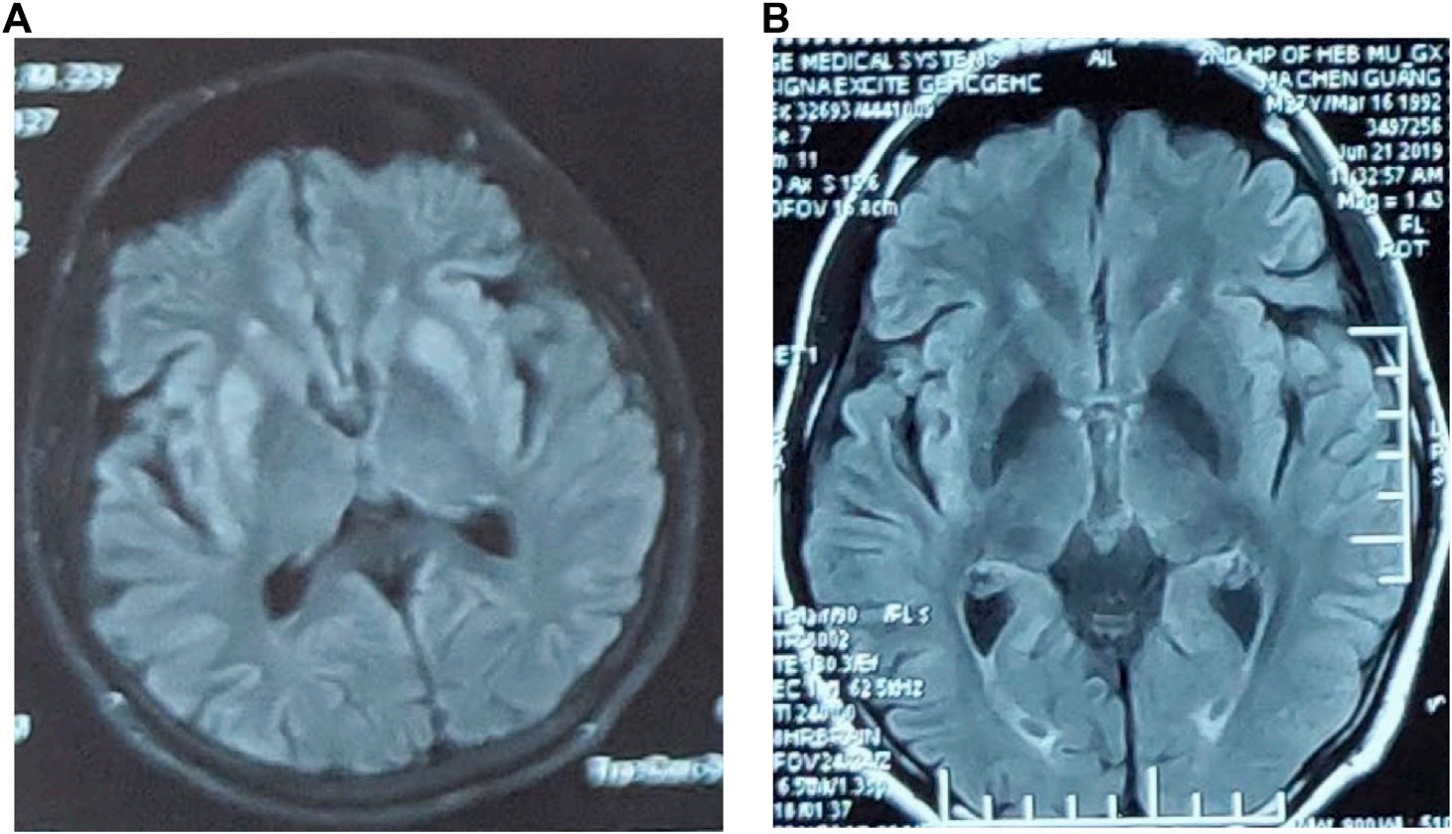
(A) Brain MRI showed symmetrical abnormal signals in bilateral basal ganglia. (B) The abnormal signal in the bilateral basal ganglia in the brain MRI disappeared.
